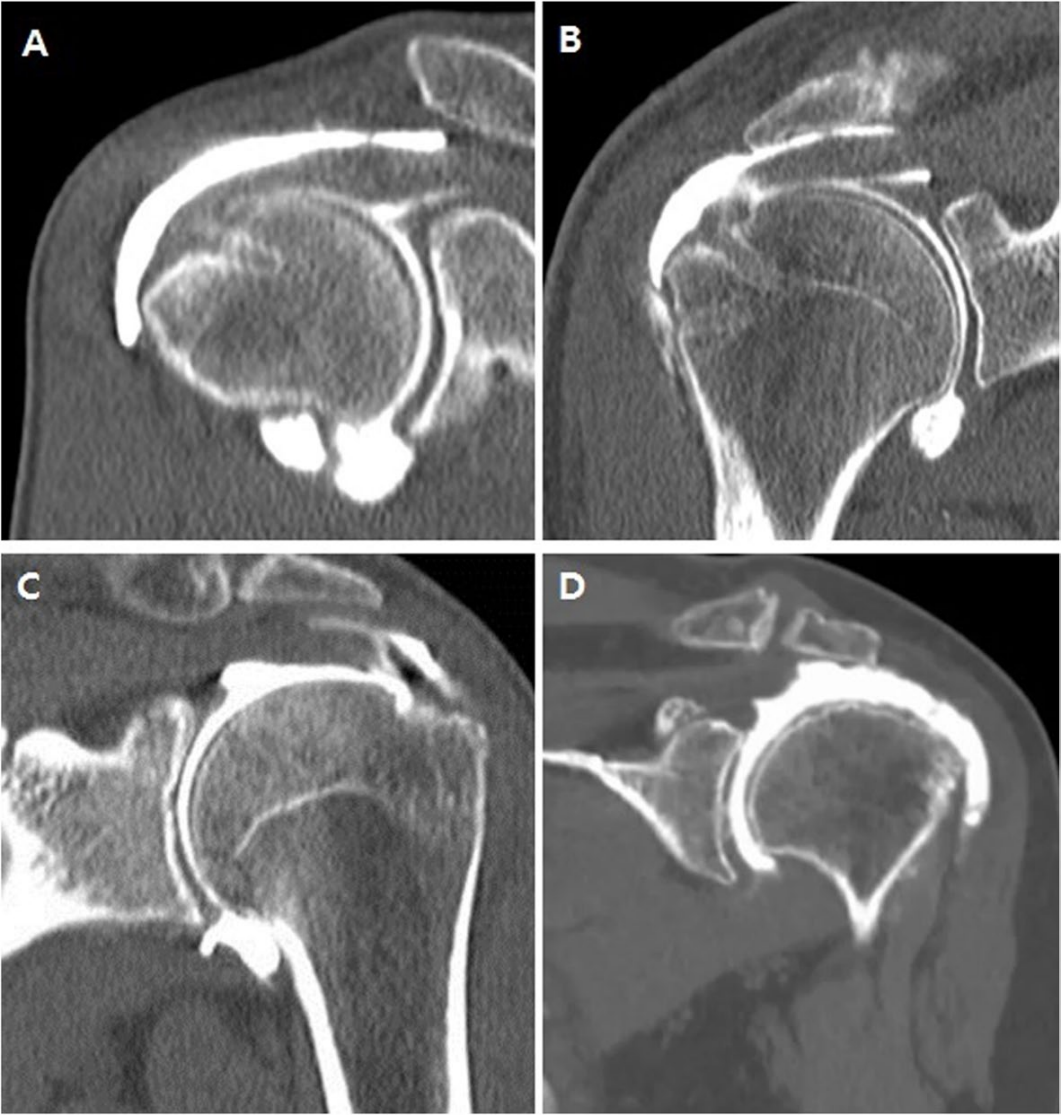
According to our CT arthrography repaired rotator cuff classification system, (A) is type Ia (footprint: full attachment, medial integrity: intact), (B) is type Ib (footprint: full attachment, medial integrity: dye leakage), (C) is type IIb (footprint: partial attachment, medial integrity: dye leakage) and (D) is type III (footprint retear)
